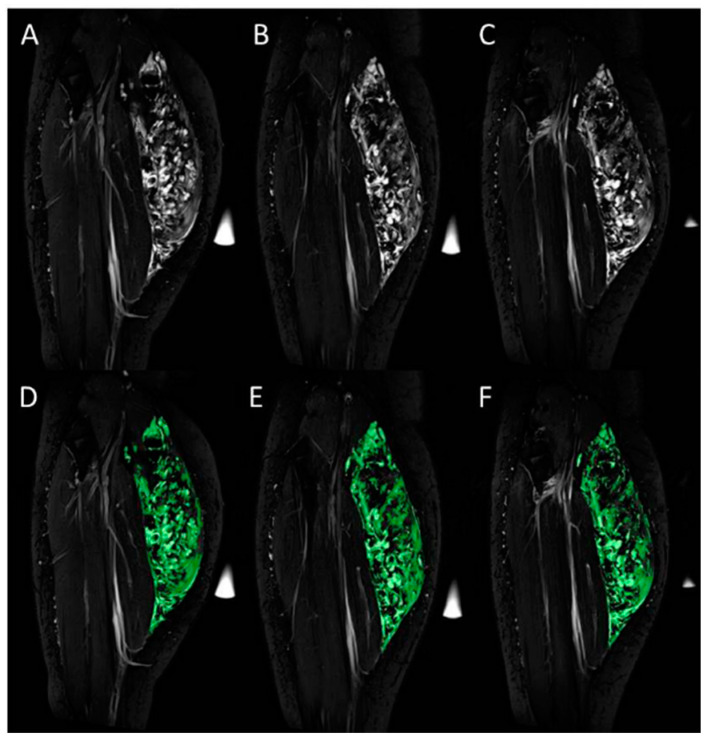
Volume of venous malformations was assessed in the T2-weighted TIRM images acquired without (A,D) and with wearing compression stockings of class I (B,E) and class II (C,F). Exemplary images before (A–C) and after color-coded manual segmentation of the venous malformation within the medial head of the gastrocnemius muscle are shown (D–F).
